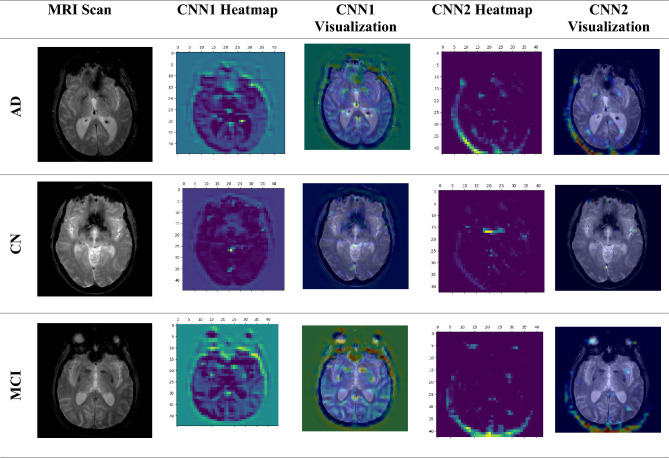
GRAD-CAM algorithm when apply to MRI scan images of an AD, CN, and MCI.
